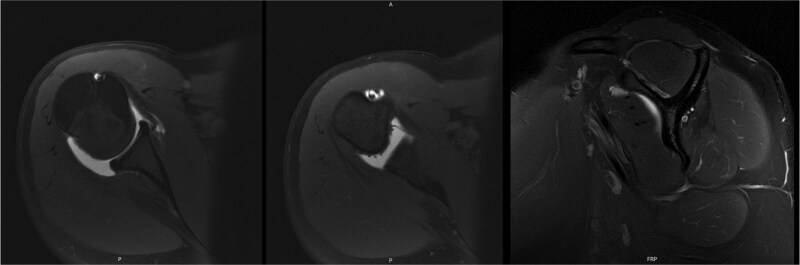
MRI at 9.5 years after decompression demonstrated increased right infraspinatus muscular tissue.
